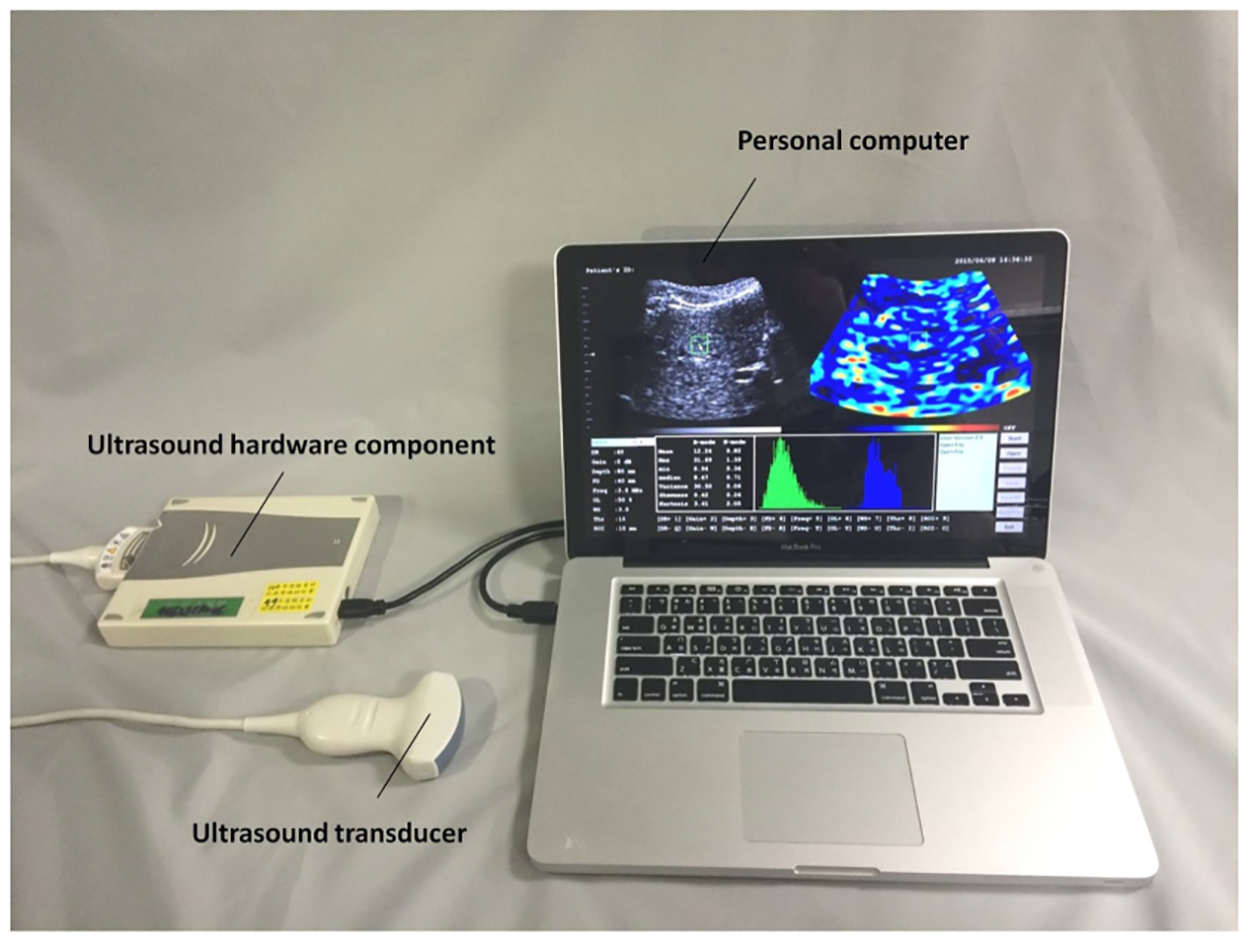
Images of the system and API. A portable clinical ultrasound scanner (Terason 3000) was used as the system platform for developing a real-time Nakagami imaging system. The software development kit provided by the manufacturer was used for system control, interface communication, and data access. A keyboard shortcut function was created to allow users to acquire image data using different central frequencies in one second.
